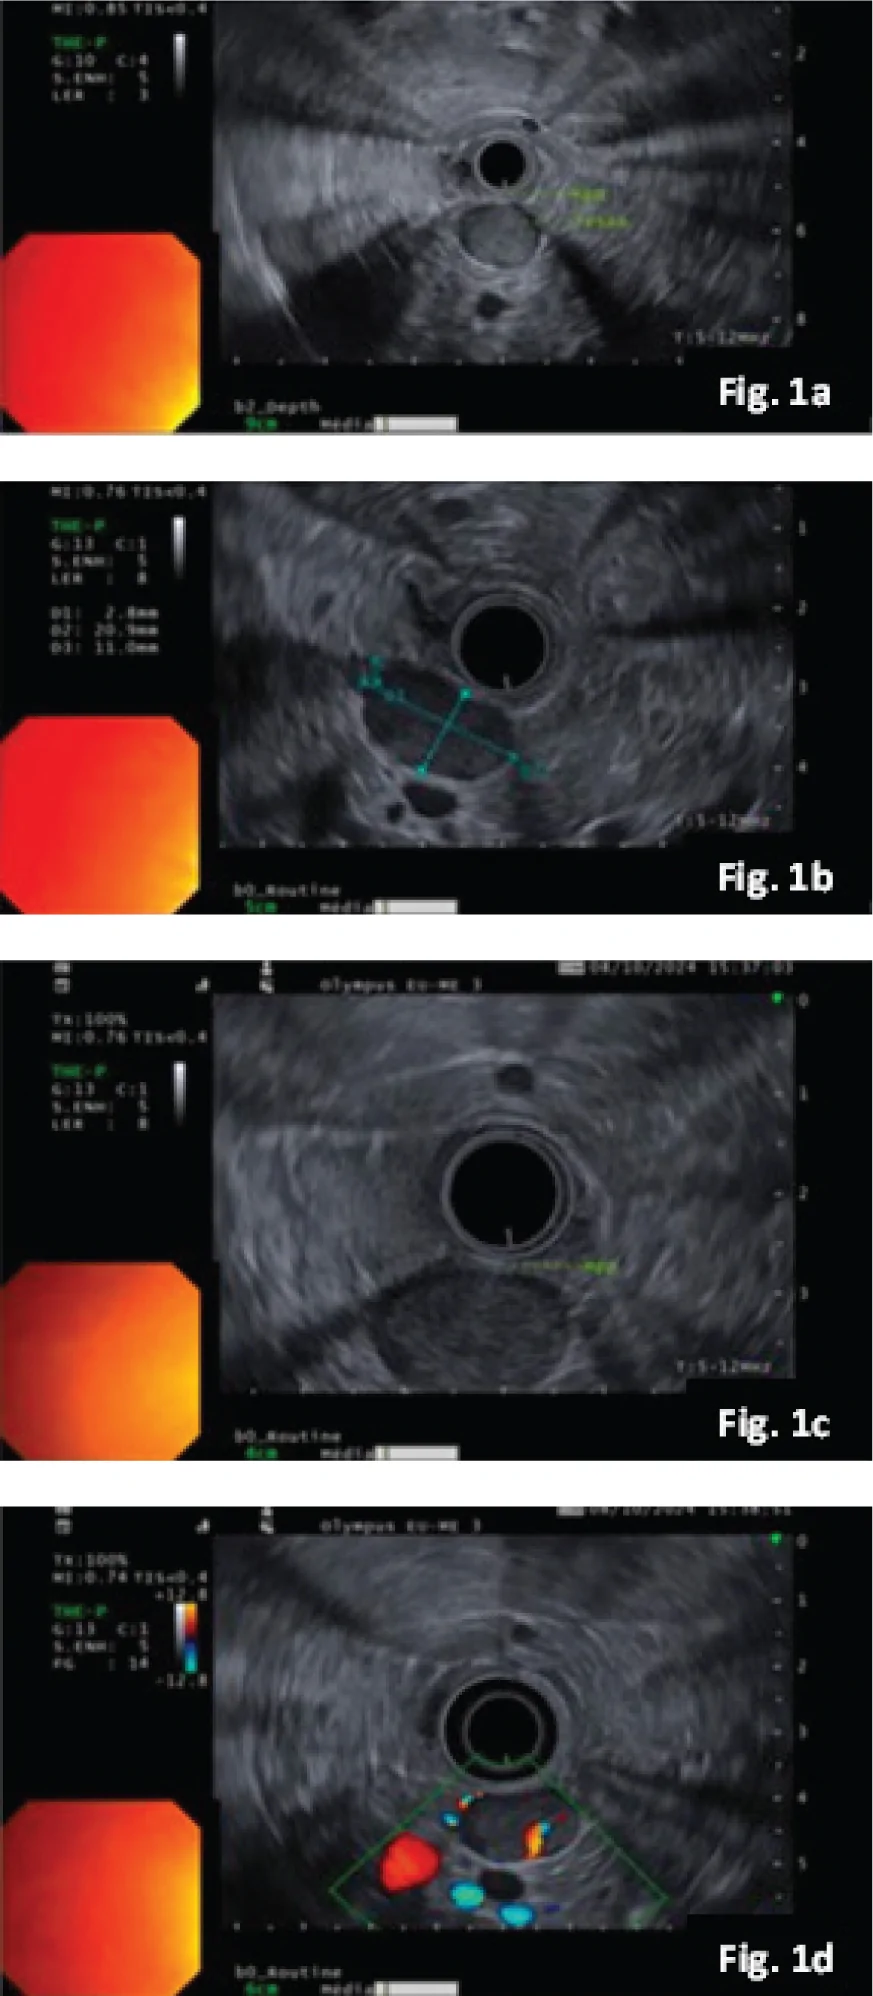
Endoscopic ultrasound (EUS) of the pancreas. (a–d) Linear-array EUS images demonstrating multiple well-demarcated, hypoechoic, solid pancreatic nodules in the body and tail, consistent with multifocal pancreatic neuroendocrine tumors. Surrounding vascular structures were preserved. Fine-needle aspiration confirmed a well-differentiated neuroendocrine tumor with a Ki-67 index < 3%.
